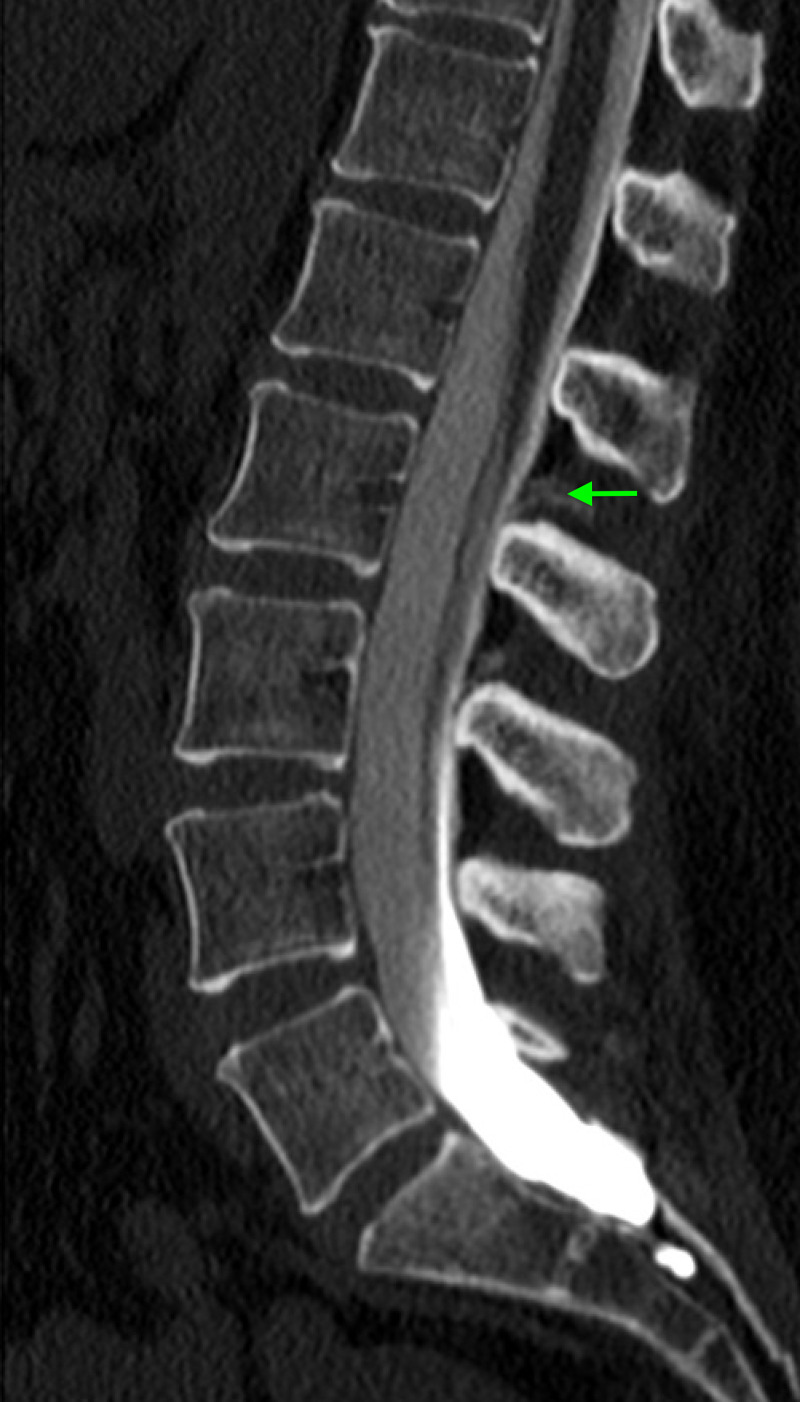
CT myelogram of the lumbar spine revealing extradural contrast extravasation within the interspinous soft tissue at L1-L2 with additional spread between the right L2-3 and L3-4 nerve roots.
